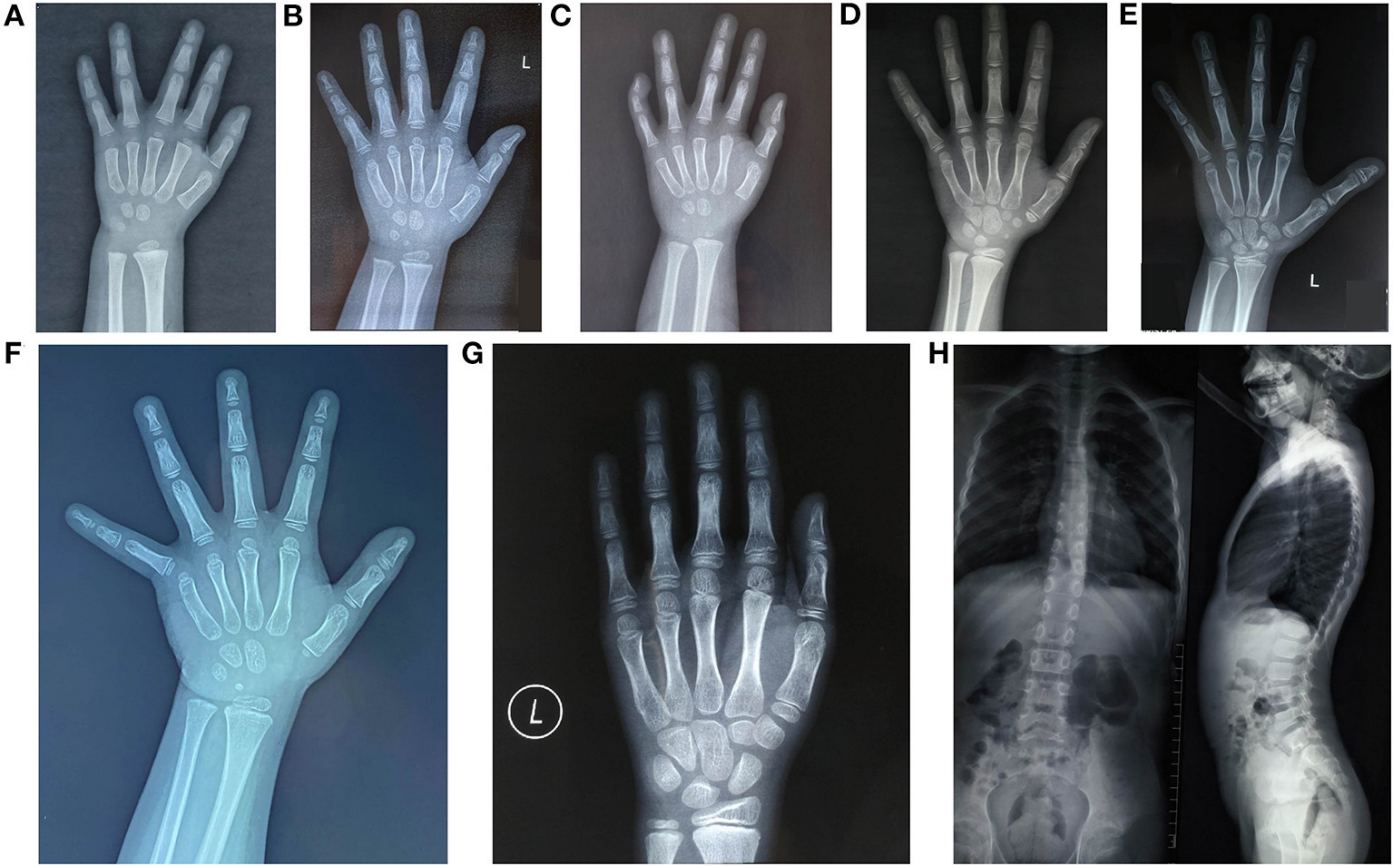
Radiology imaging data of the seven patients studied. (A) Patient 1, BA 2.6 years old, CA, 3 years old; (B) Patient 2, BA, 3.6 years old, CA, 3.1 years old, with a short thumb; (C) Patient 3, BA, 2 years old, CA, 2.9 years old, the 5th distal phalanx bended inward; (D) Patient 4, BA, 8.2 years old, CA, 7.3 years old; (E) Patient 5, BA, 10 years old, CA, 9.4 years old, with a short thumb; (F) Patient 6, BA, 3.8 years old, CA 3.4 years old, with a short thumb; (G, H) Patient 7, the left-hand X-ray showed BA, 10.2 years old and CA, 9.1 years old; spine lateral X-ray revealed mild thoracolumbar scoliosis. P, proband; BA, bone age; CA, age.
